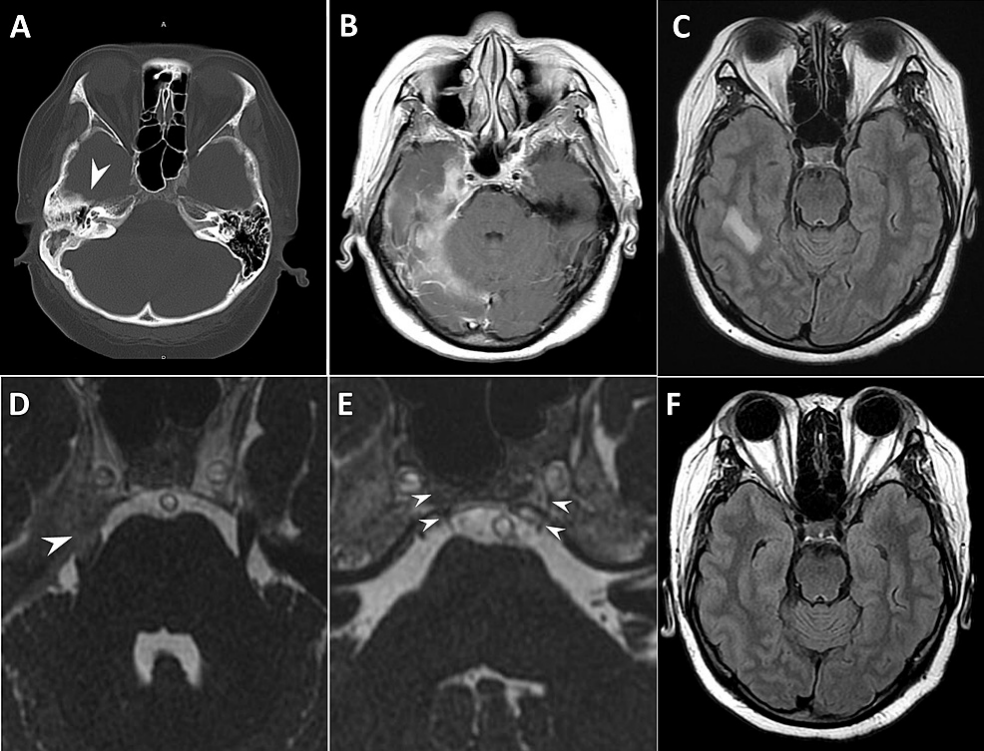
Brain computed tomography (CT) scan and magnetic resonance imaging (MRI) results indicated Gradenigo’s syndrome, which was characterized by pachymeningitis, leptomeningitis, and cerebritis. Non-contrast-enhanced brain CT scan showed right petrous apicitis with ill-defined irregular edges, and there was soft tissue in the mastoid cavity and less pneumatized mastoid air cell system (arrowhead) as compared to the opposite side suggesting chronic otitis media. (A). Brain MRI revealed pachymeningitis, leptomeningitis, and cerebritis involving the right tentorium cerebelli (B) and the right temporal region (C). Moreover, there was inflammation in the Meckel cave and CN V (arrowhead) (D). The right Dorello canal was swollen compared with the left one (arrowheads) (E). Follow-up MRI revealed the complete resolution of previous signal abnormalities. Cerebritis in the right temporal area [(F) vs (C)].
